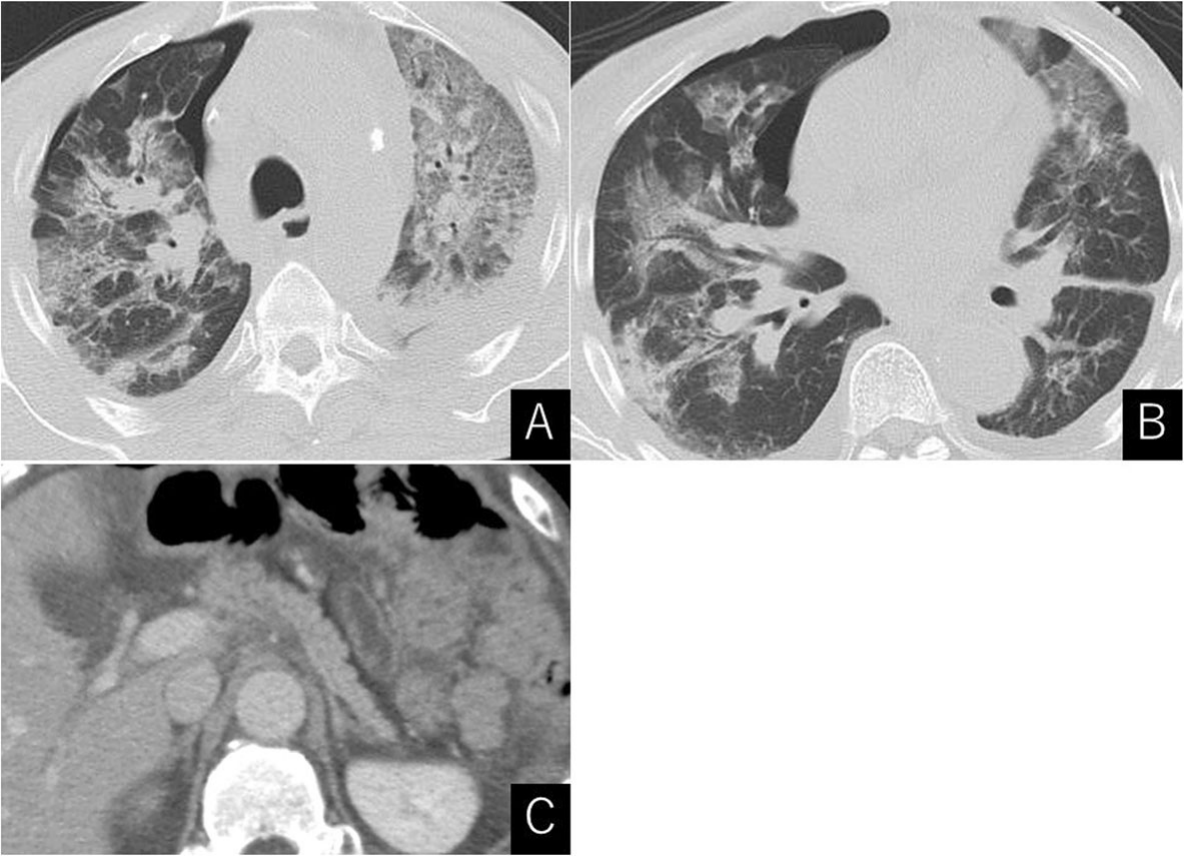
CT scan showed severe bilateral pulmonary edema. Small amount of iatrogenic pneumothorax was secondary to thoracentesis (a, b). Pancreas was intact (c)
